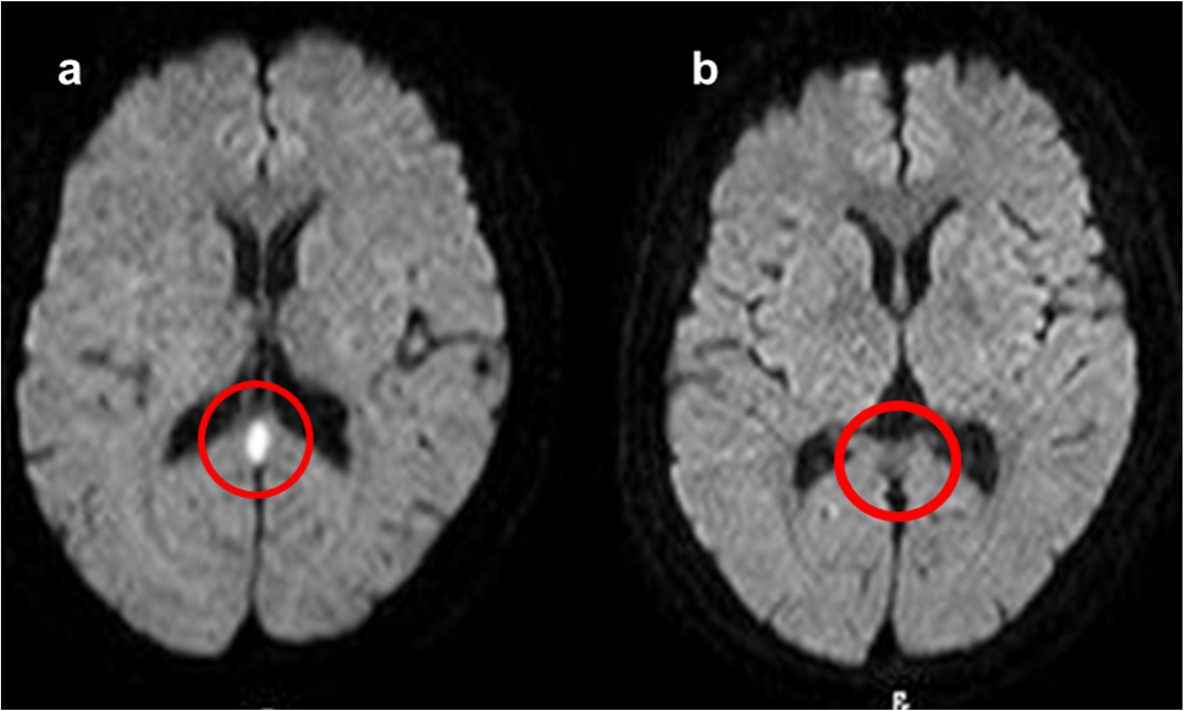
Head magnetic resonance imaging (MRI). a Diffusion-weighted imaging on admission day 2, b Diffusion-weighted imaging on admission day 9. Abnormalities detected on MRI completely disappeared on admission day 9 (red circles)
